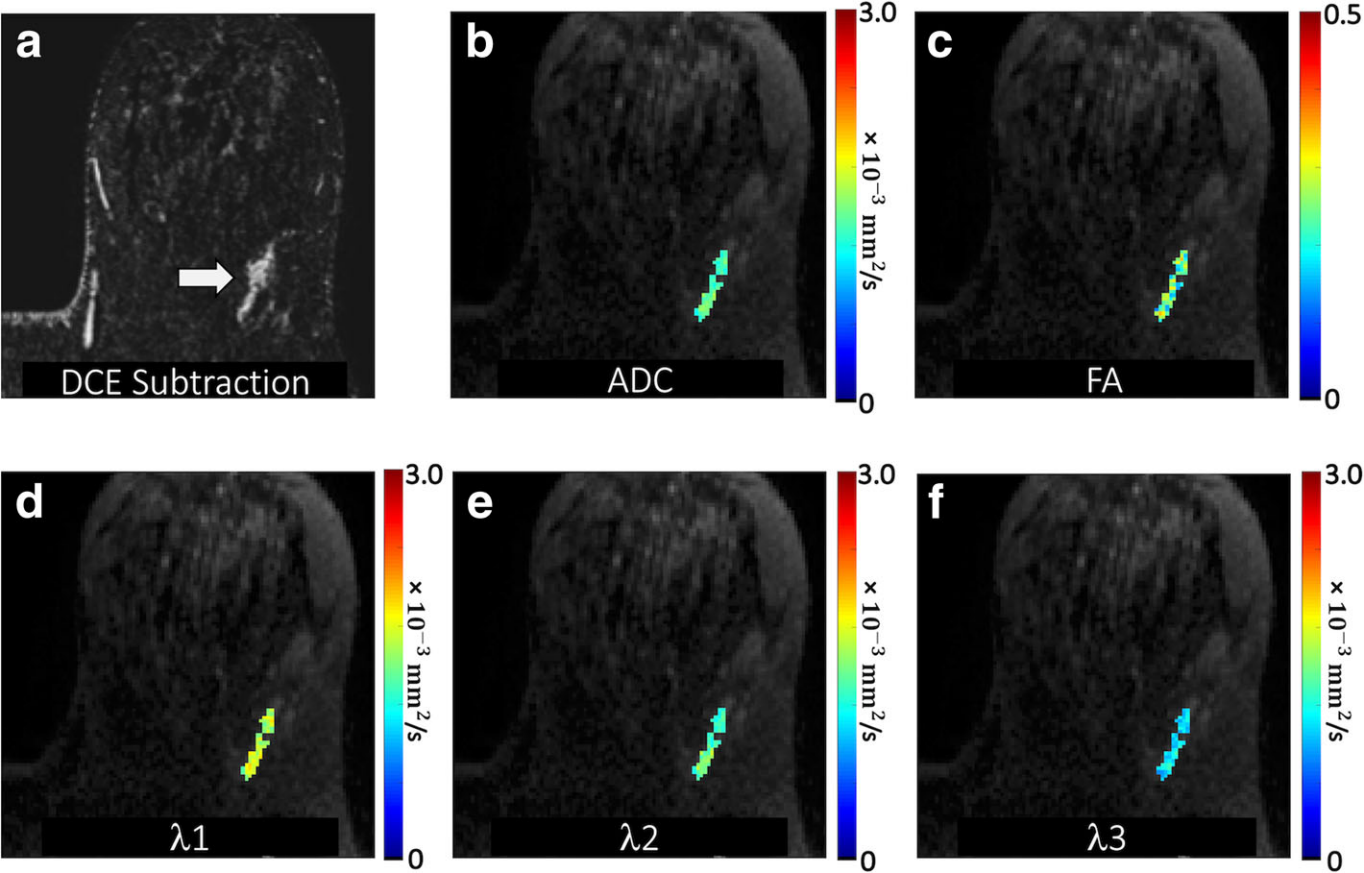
Malignant non-mass enhancement detected in a 58-year-old patient undergoing MRI for high risk screening. a DCE post-contrast image demonstrates a 33-mm linear heterogeneous non-mass enhancement in the posterior left breast 2 o’clock (arrow), assigned a BI-RADS category 4. DTI-derived parametric maps of b apparent diffusion coefficient (ADC), c fractional anisotropy, and eigenvalues d
